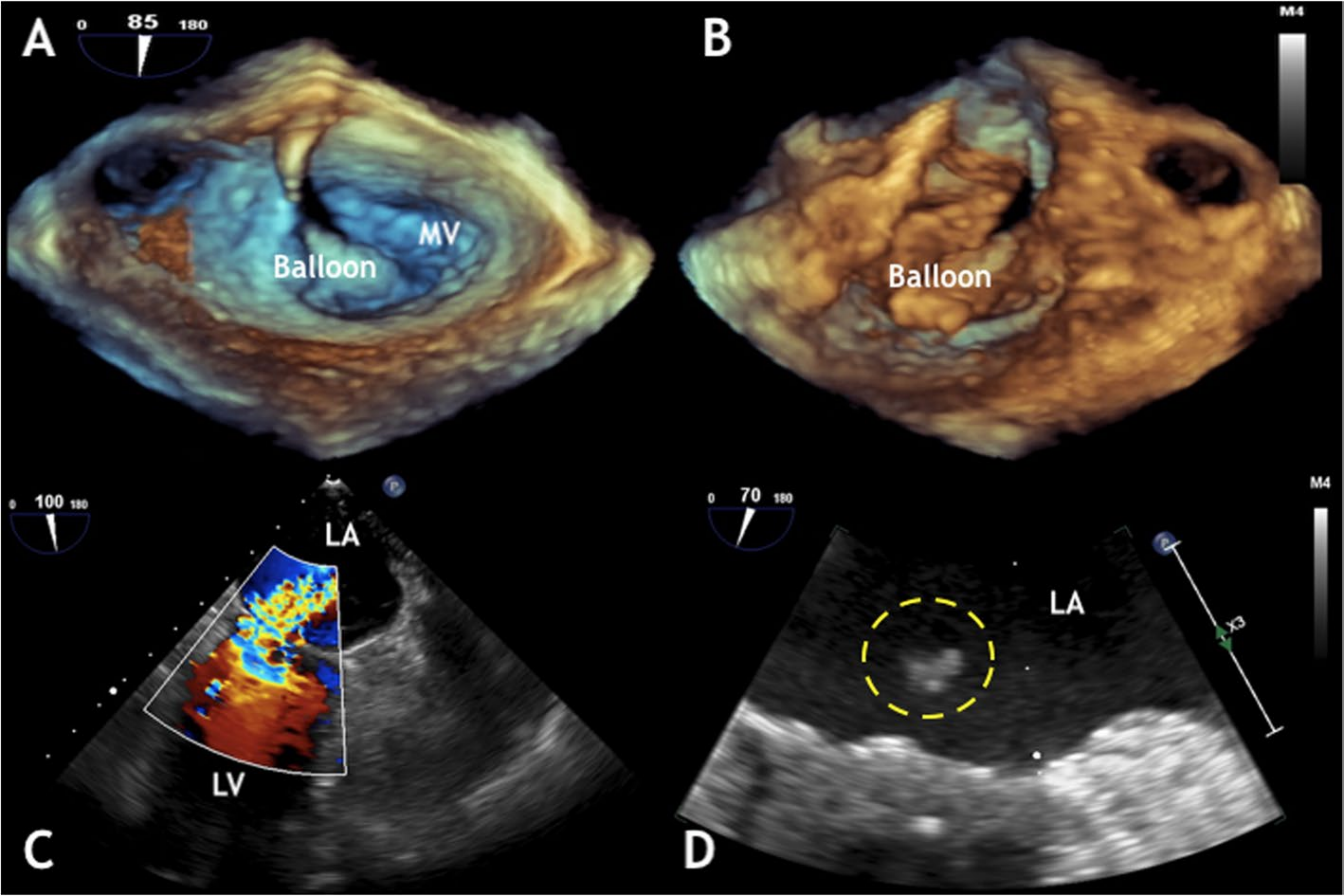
Intra-operative Transesophageal Echocardiographic imaging of the Percutaneous Mitral Balloon Valvuloplasty (PMBV). A Three-dimensional reconstruction demonstrating the balloon through the mitral valve (MV) coming from the left atrium, and the same view is seen from the left ventricle in B. C Immediate severe mitral regurgitation jet from the left ventricle (LV) into the left atrium (LA). D Piece of mitral chordae into the LA (yellow circle)
